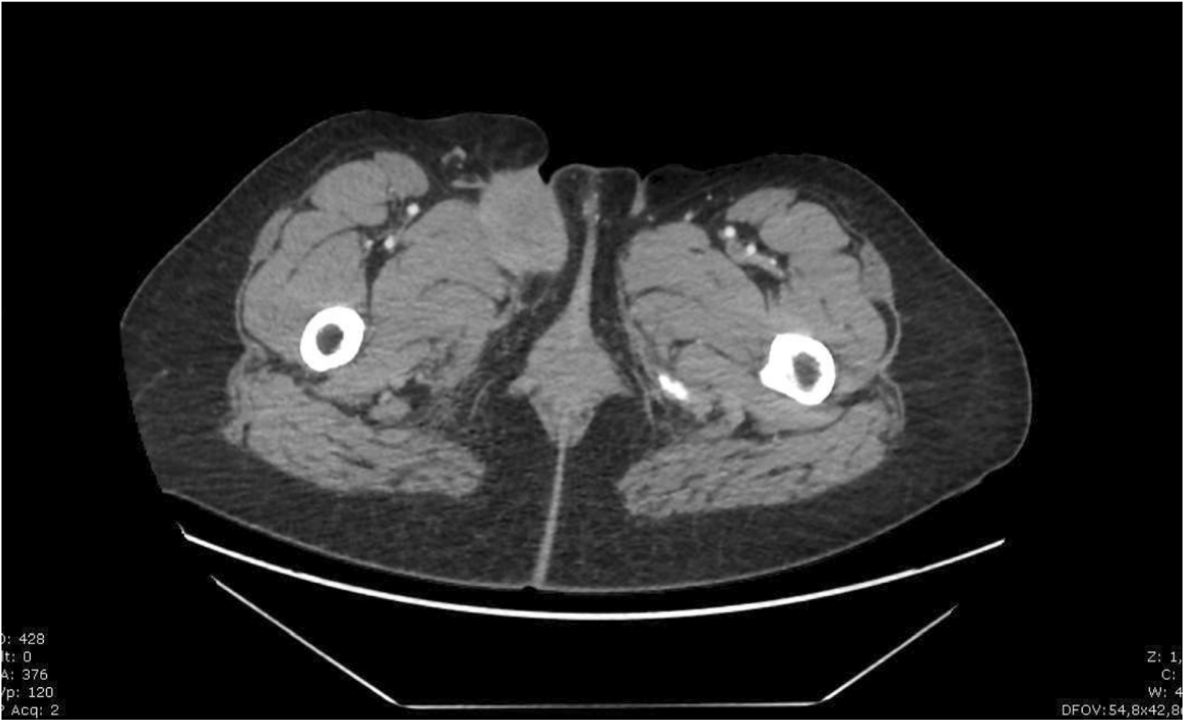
Initial CT scan. Axial CT scan showing an inguinal non-circumscribed contour 5 cm long axis, mass adhering to skin and in contact with the adductor muscle of the deep lip
